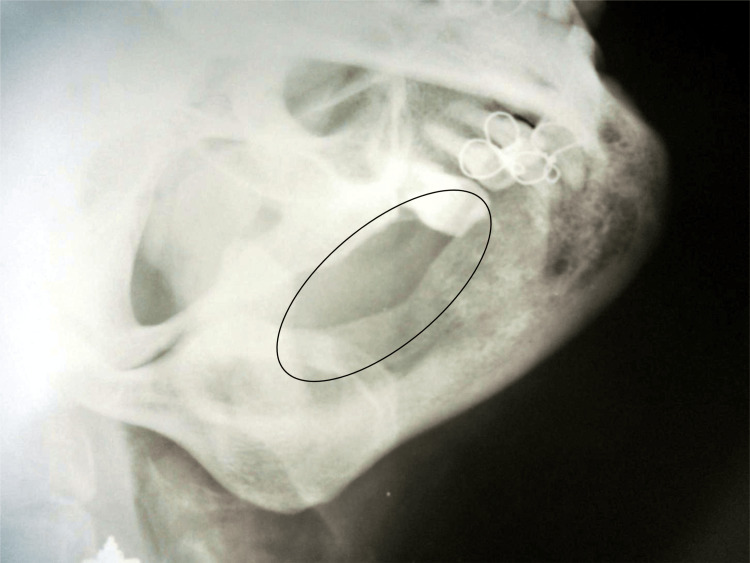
Follow-up left lateral oblique ramus-body projection radiograph after six months to inspect for recurrence of the lesion. There was no evidence of recurrence of the lesion
